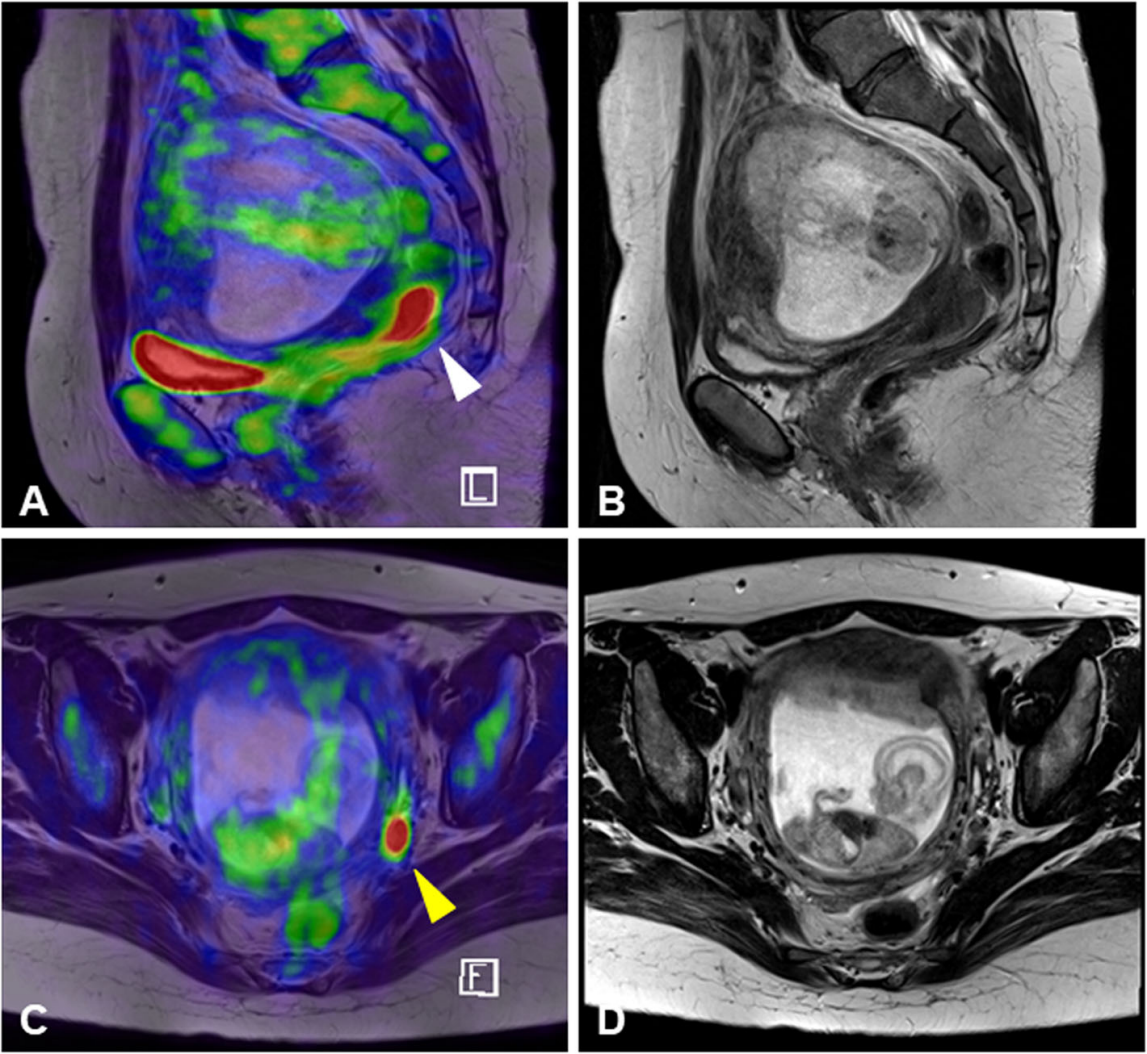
PET/MR images of case #6. a Sagittal views of PET/MR fusion image show increased FDG uptake of cervical tumor (white arrowhead). b T2-weighted magnetic resonance image corresponding to PET/MR image (a). c Axial views of PET/MR fusion image show increased FDG uptake of pelvic lymph node (yellow arrowhead). d T2-weighted magnetic resonance image corresponding to PET/MR image (c)
